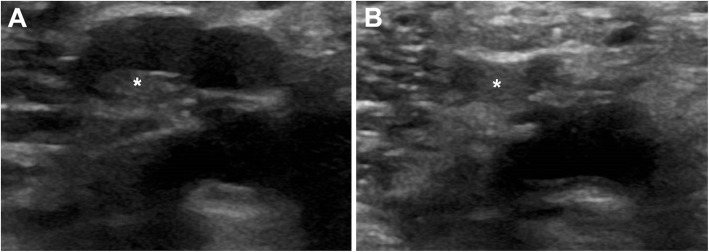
Screening ultrasound. The images display the bifurcation of the popliteal vein into the calf veins. The thrombus, marked with an asterisk (*), can be distinguished even without compression in the left vein (a). The left vein cannot be fully compressed, which is diagnostic of thrombosis, whereas the right vein demonstrates normal compression with full collapsibility (b). This pathological screening ultrasound was later confirmed by formal ultrasound
